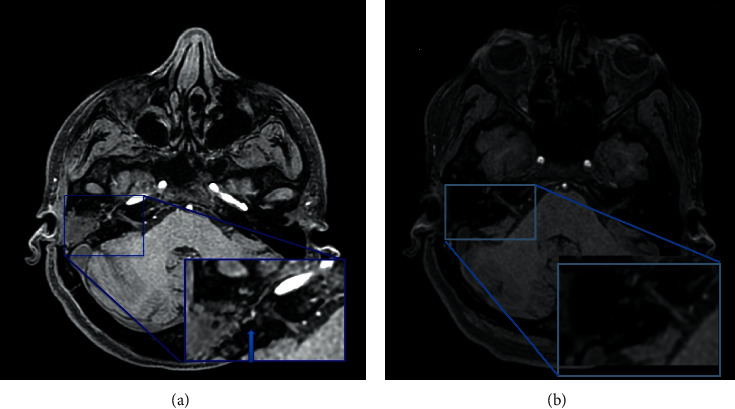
(a) Axial T1 fat-saturated magnetic resonance imaging scan of the middle ear; blue arrow indicated the residual tumor in the middle ear before the canal-wall-down tympanoplasty. (b) Axial T1 fat-saturated magnetic resonance imaging scan of the middle ear after one year from the surgical procedure; the blue rectangle showed the absence of recurrence or persistence of the disease.
